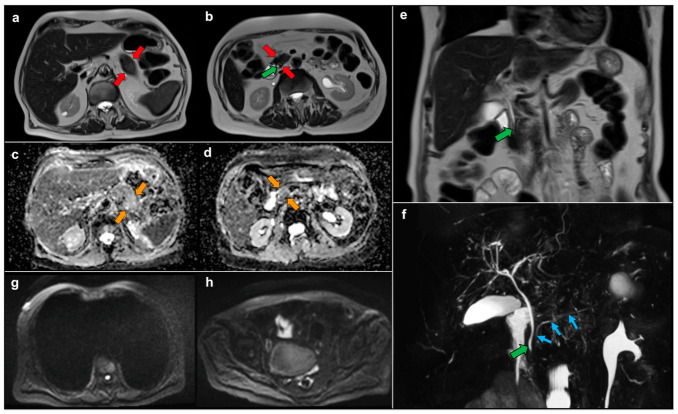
Follow-up MRI of the previous patient (IgG4-RD with multifocal AIP and multiorgan involvement) performed after 2 months of steroid therapy. (a,b,e) Axial and coronal T2WI, (c,d) ADC maps and (f) Coronal 3D MRCP with MIP reconstruction demonstrate marked improvement of the pancreatic fibroinflammatory changes (red arrows), with increased ADC values (orange arrows), as well as resolution of the MPD stricture and mild upstream dilatation (blue arrows). Note also the marked regression of the stenosis and circumferential thickening of the distal CBD (green arrow), without upstream biliary dilatation. (g,h) DWI (b = 800 s/mm^2^) shows near-complete resolution of the previously observed areas of restricted diffusion in the pleura and presacral fat.
